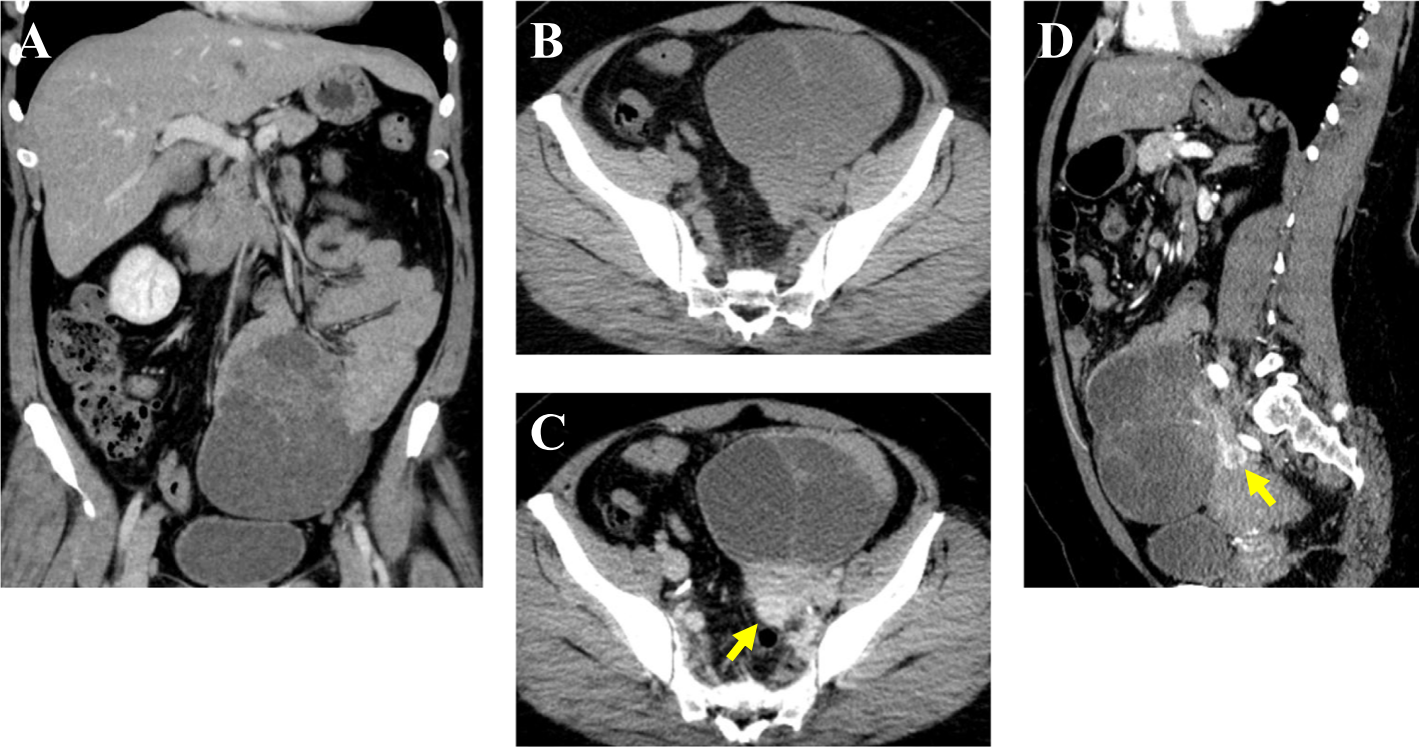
Abdominal and pelvic CT findings. A Coronal image. The multicystic mass changed in position to the caudal direction in comparison with the MRI findings, and a jejunal origin was suggested. No dilatation of the intestine or intraluminal component was found. B Plain image of the tumor. C Contrast-enhanced image of the tumor. The multicystic tumor showed a thin wall and multiple septations, with low-density liquid showing no enhancement and an enhanced solid compartment. The thickened portion of the left ventral region is the jejunum. On the back side of the solid compartment, there was a round solid nodular mass, measuring 18 mm in maximal diameter, showing strong enhancement in the early period of the contrast-enhanced sequence, unlike the cystic tumor component. D Sagittal contrast-enhanced CT image. The solid nodular mass showed a heterogeneous rim-like enhancement pattern with attenuated central areas, unlike the other cystic or solid compartments of the tumor
